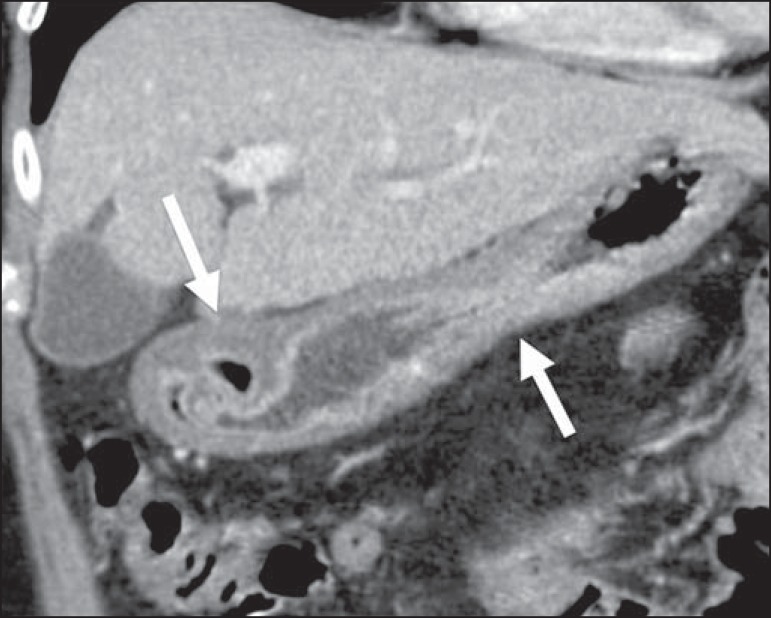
Gastric lymphoma. Contrast-enhanced CT of the abdomen, in coronal
